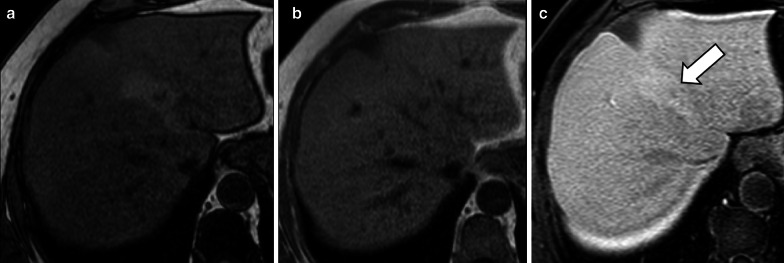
A 63-year-old man with liver steatosis and focal fat sparing area in segment 4. Gadoxetate disodium-enhanced MRI shows (a) in the opposed-phase and (b) in-phase images a fat sparing area with similar signal unlike the background liver that has marked signal drop in the opposed-phase sequence. The area of fat sparing is (c) slightly hyperintense (arrow) to the background liver in the hepatobiliary phase
